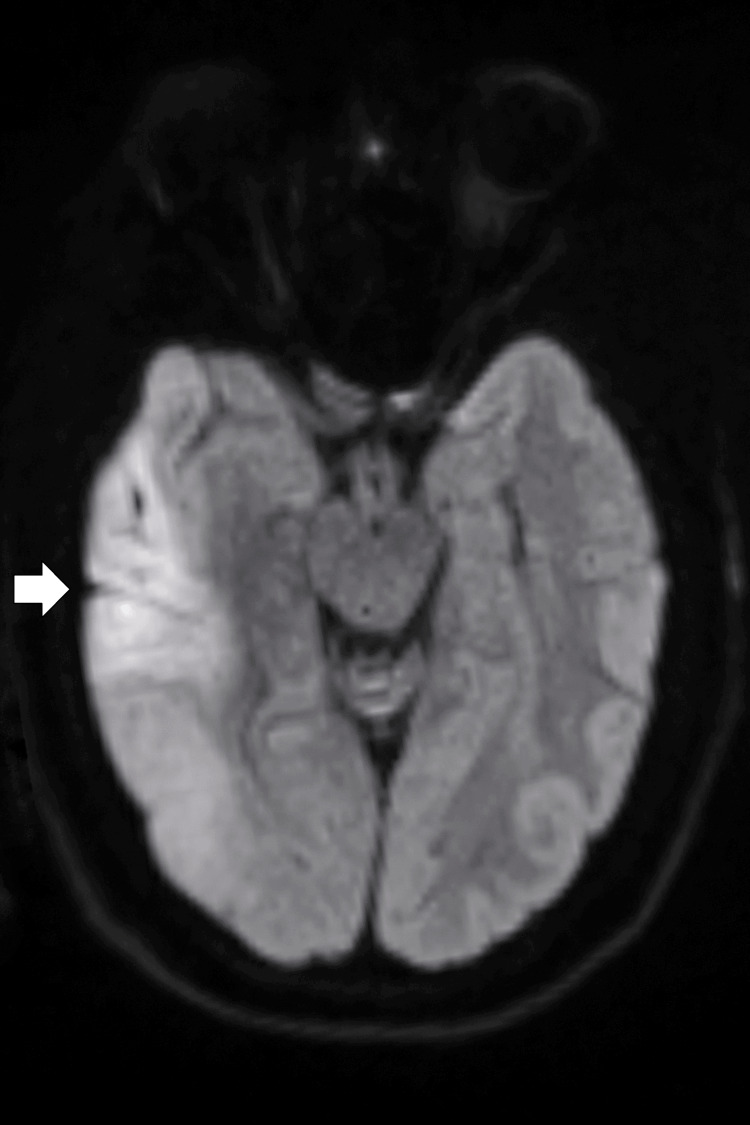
Axial DWI MRI of the brain Axial DWI MRI demonstrating a focal area of cortical diffusion restriction in the right temporal lobe (white arrow), extending into the adjacent subcortical white matter. The lesion appears hyperintense relative to the surrounding parenchyma, without associated midline shift or mass effect. Findings are in keeping with acute cortical involvement, initially suggestive of ischemia but subsequently attributed to familial hemiplegic migraine. MRI: magnetic resonance imaging; DWI: diffusion-weighted imaging
